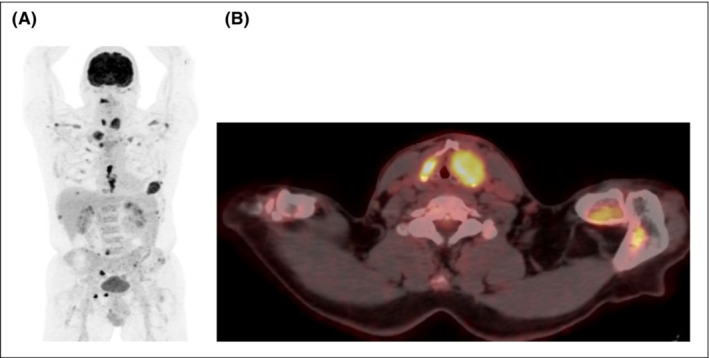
18‐FDG PET‐CT. Multiple foci of strongly FDG‐avid lesions can be observed throughout the skeleton and bilateral in the thyroid cartilage (L > R). No other zones with increased tracer activity can be detected. (A) Coronal view of maximum intensity projection. (B) Axial cervical section of PET‐CT.
